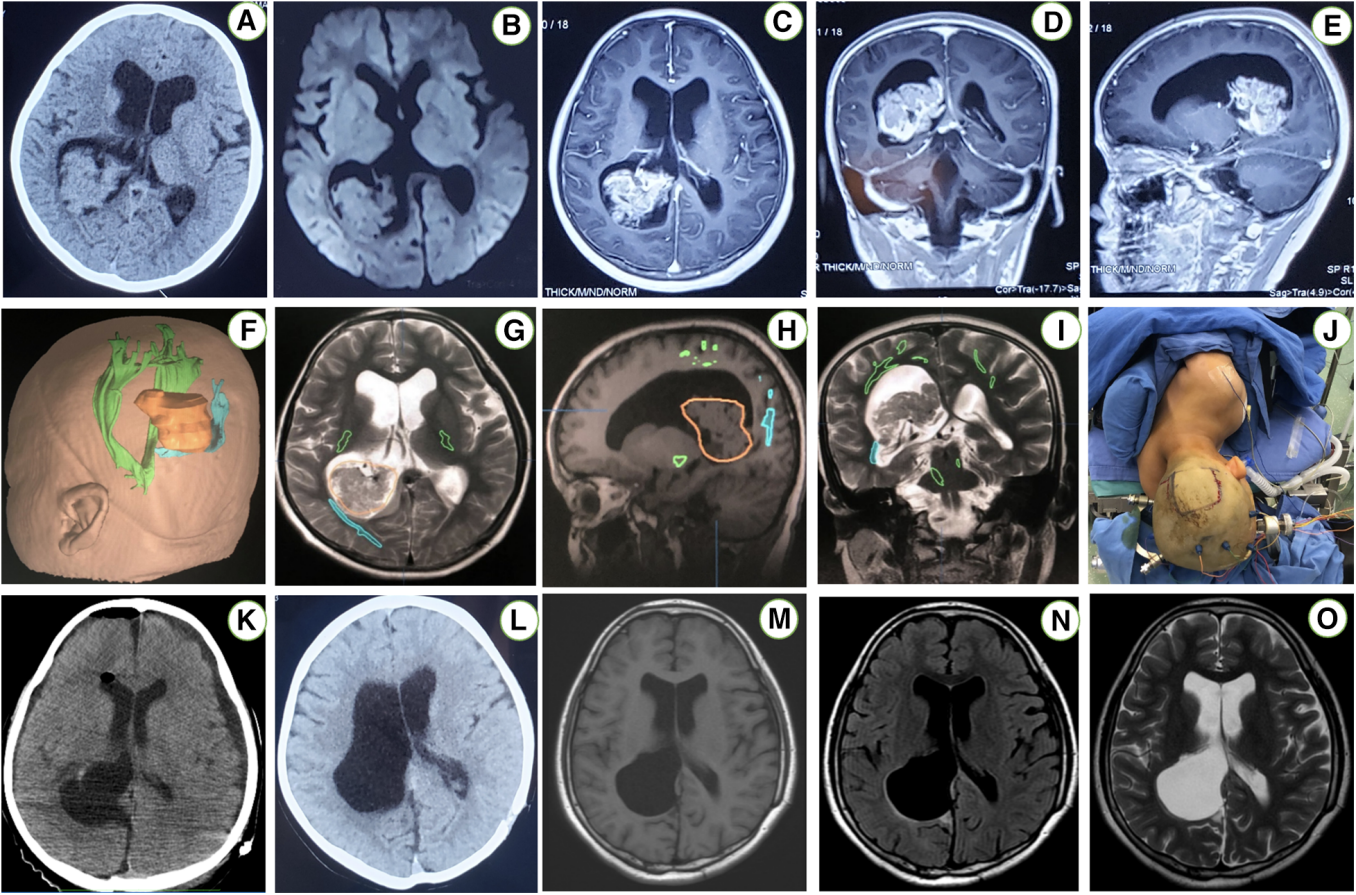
An axial CT scan of the head (A) reveals a right periatrial space occupying lesion. An axial T1-weighted MRI without contrast (B) demonstrates the exact location of the periatrial tumor. Enhanced images in sagittal, coronal, and transverse views of this patient brain MRI (C–E) demonstrated berry-like and irregular enhanced lesions in the right trigone of the lateral ventricle. Registration of the image-guided system (F–I) was performed. The patient position (J) in the contralateral PITTA. An axial postoperative CT scan (K) reveals a right atrial CPP that was resected via the left PITTA. An axial MRI T1-weighted sequence without contrast (L) excludes any unexpected injury to either posterior hemisphere. Postoperative axial T1-flair, T2-flair and T2-weighted without contrast images 4 years later (M–O) demonstrated complete resection via a contralateral approach.
